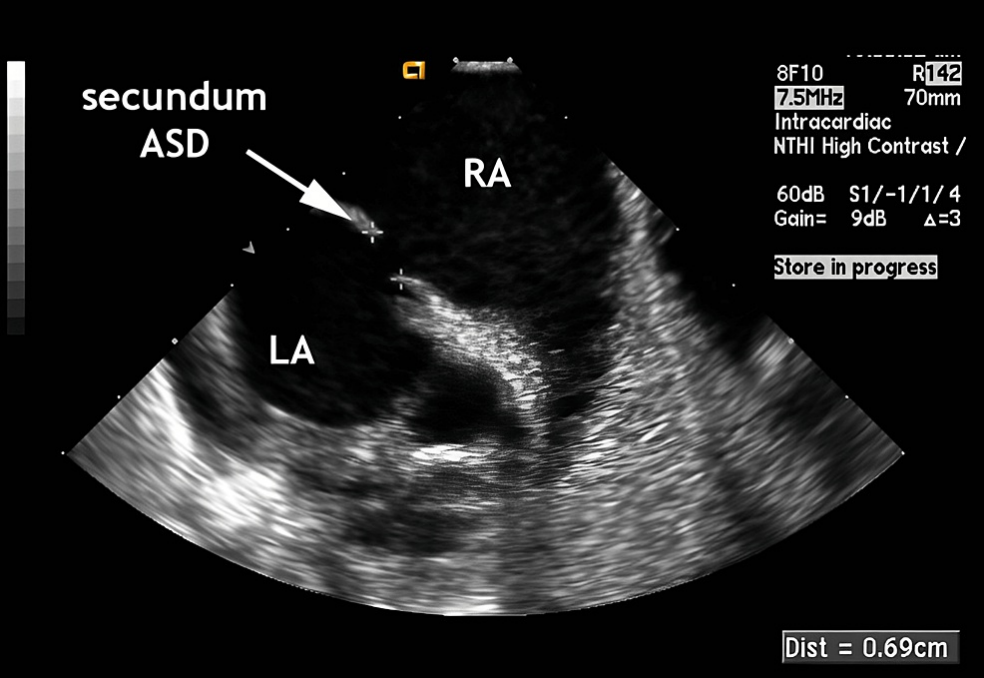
Transesophageal echocardiogram (case 1) showing interatrial communication, ostium secundum type, with 7 mm of diameter and presenting a bidirectional shunting. ASD - Atrial septal defect; LA - Left atrium; RA - Right atrium
